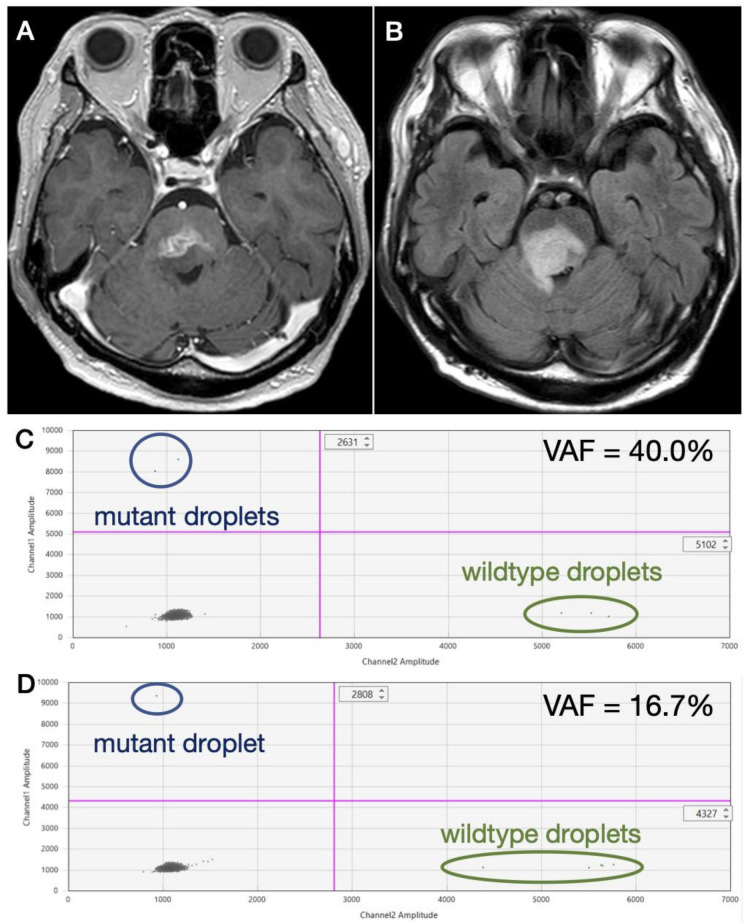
A recurrent DMG case in which *H3F3A* K27M was suspected. MR images show (A) a heterogeneously enhancing lesion with possible necrosis and (B) perifocal hyperintensity on fluid attenuated inversion recovery (FLAIR). Since mutant droplets were detected from both wells by ddPCR analysis, *H3F3A* K27M mutation was strongly considered. However, since only (C) two and (D) one mutant droplets were detected from each well, a definitive diagnosis was not achieved.
